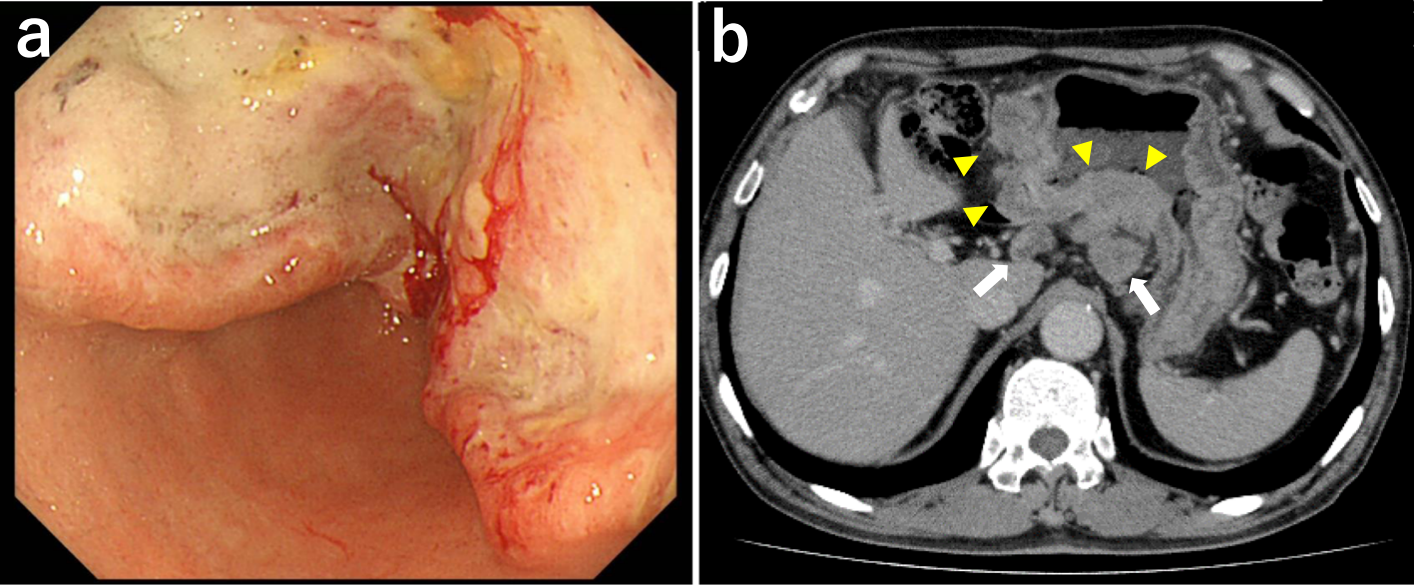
Imaging before combined therapy. a Gastroscopy showed a large Borrmann type II lesion in the middle and distal stomach. b Contrast-enhanced computed tomography scan showed thickening of the wall of the stomach (arrowhead) and enlarged regional lymph nodes (arrow)
